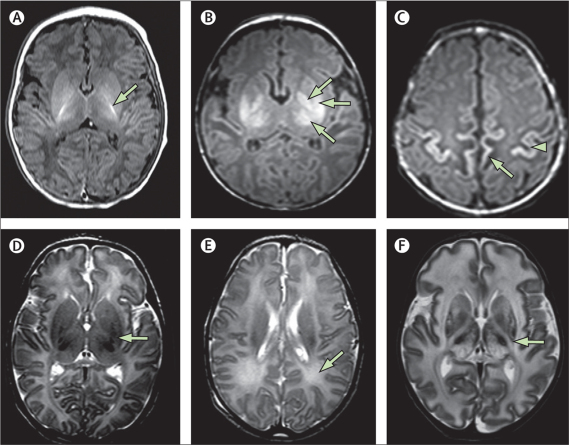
MRI appearances in neonatal hypoxic–ischaemic encephalopathy (A-C) T1-weighted images in the transverse plane. (A) Normal neonatal brain with linear high signal intensity representing myelin in the posterior limb of the internal capsule (arrow). (B) Moderate basal ganglia and thalamic lesions with abnormal increased signal intensity in the globus (top arrow), putamen (middle arrow), and thalamus (bottom arrow). There is no normal linear high signal intensity from the intervening posterior limb of the internal capsule. (C) Cortical lesions. There is abnormal increased signal intensity in the cortex around the central sulcus (arrow head) and along the interhemispheric fissure. Abnormal low signal intensity is seen in the adjacent subcortical white matter (arrow). (D–F) T2-weighted images in the transverse plane. (D) Mild lesions in the basal ganglia. There are small bilateral foci of abnormal low signal intensity in the lentiform nuclei (arrow). (E) Mild white matter lesions. There is diffuse and slightly increased signal intensity in the periventricular white matter (arrow). (F) Severe basal ganglia and thalamic and white matter lesions. There is mixed abnormal signal intensity throughout the basal ganglia and thalami. The intervening posterior limb of the internal capsule shows abnormal high signal intensity with no evidence of low signal intensity from myelin (arrow). The hemispheric white matter has diffuse abnormal high signal intensity throughout.
